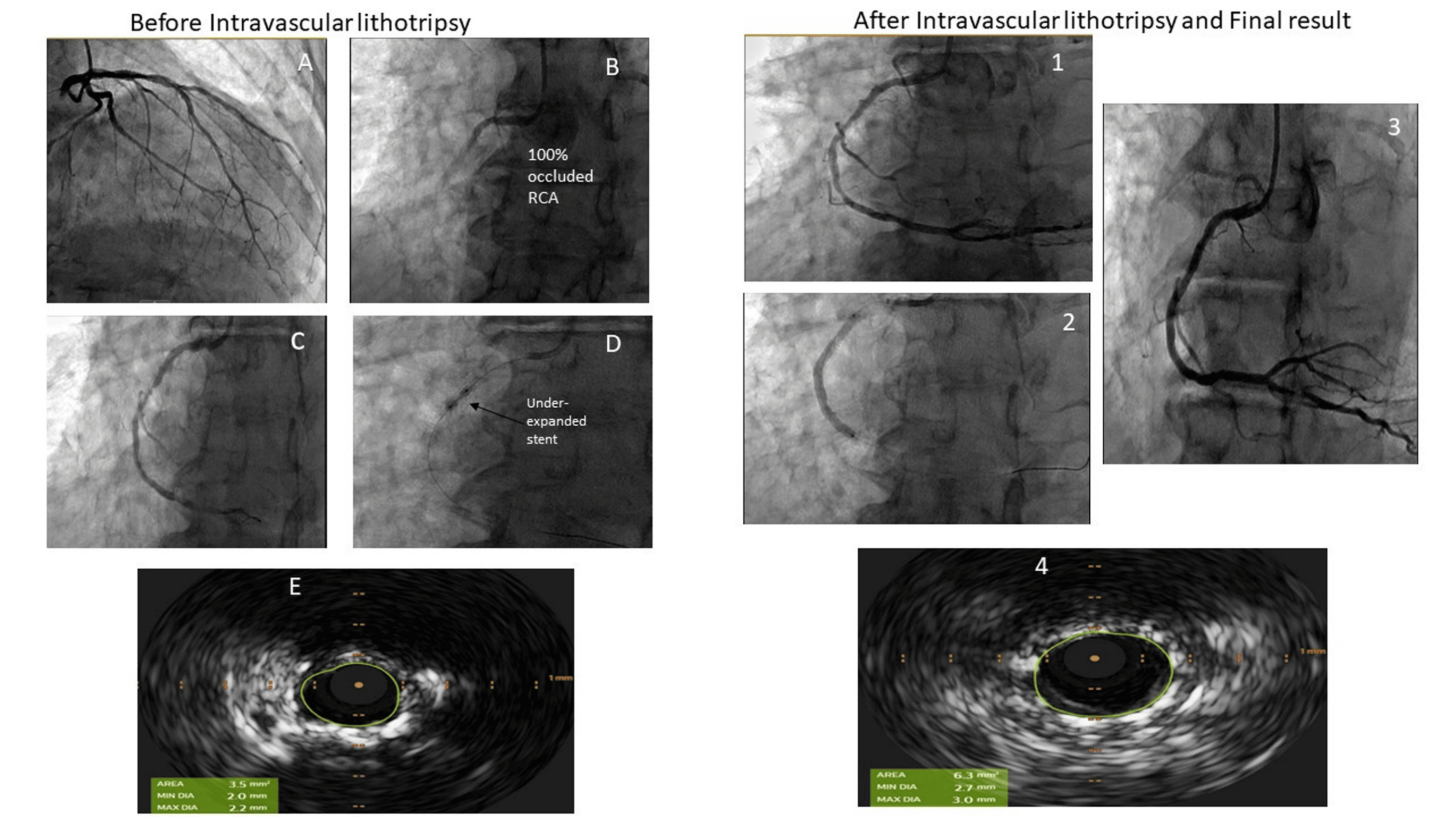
Angiogram and intravascular ultrasound pre- and post-intervention of a representative patient included in the study. Before intravascular lithotripsy A: Angiogram of the left coronary system demonstrating moderate disease in the mid-left anterior descending artery. B: Angiogram of the RCA demonstrating 100% acute occlusion in the proximal segment with TIMI 0 flow. C: RCA angiogram demonstrating 90% in-stent restenosis in the mid-segment. D: Balloon angioplasty of mid-RCA stenosis demonstrating underexpansion of the balloon. E: Intravascular ultrasound of mid-RCA lesion demonstrating severely reduced minimal stent area 3.5 mm². After intravascular lithotripsy 1: Angiogram of RCA demonstrating good expansion of lesion in the mid-RCA. 2. Deployment of the stent in the proximal and mid-RCA with good expansion 3. Final angiogram of RCA demonstrating well-expanded stent with TIMI 3 flow. 4. Intravascular imaging of RCA demonstrating good stent expansion and apposition with an increase in minimal stent area to 6.3 mm². RCA: right coronary artery
